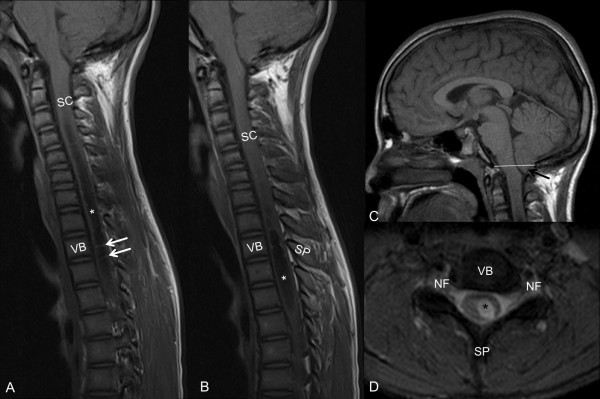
Magnetic resonance image of the proband. Sagittal SE T1-weighted cervical MRI (A, B) shows cerebrospinal fluid (CSF)-filled cavity (*) in the middle of the central cervical and thoracic spinal cord (SC), with multiple caudal septations. These non-neoplastic septated (white arrows) paracentral fluid containing cavitations are referred to as hydrosyringomyelia. Sagittal SE T1-weighted brain MRI (C) with the basion-opisthion line (BOL) shown in white. Note crowded foramen magnum and the low-lying pointed tonsil with distance from BOL >5 mm (black arrow), consistent with Chiari malformation 1. Axial MERGE T2*-weighted cervical MRI (D) shows a well-demarcated CSF cavity (*) in the middle central and left paracentral SC. VB, vertebral body; NF, neural foramina; SP, spinous process.
